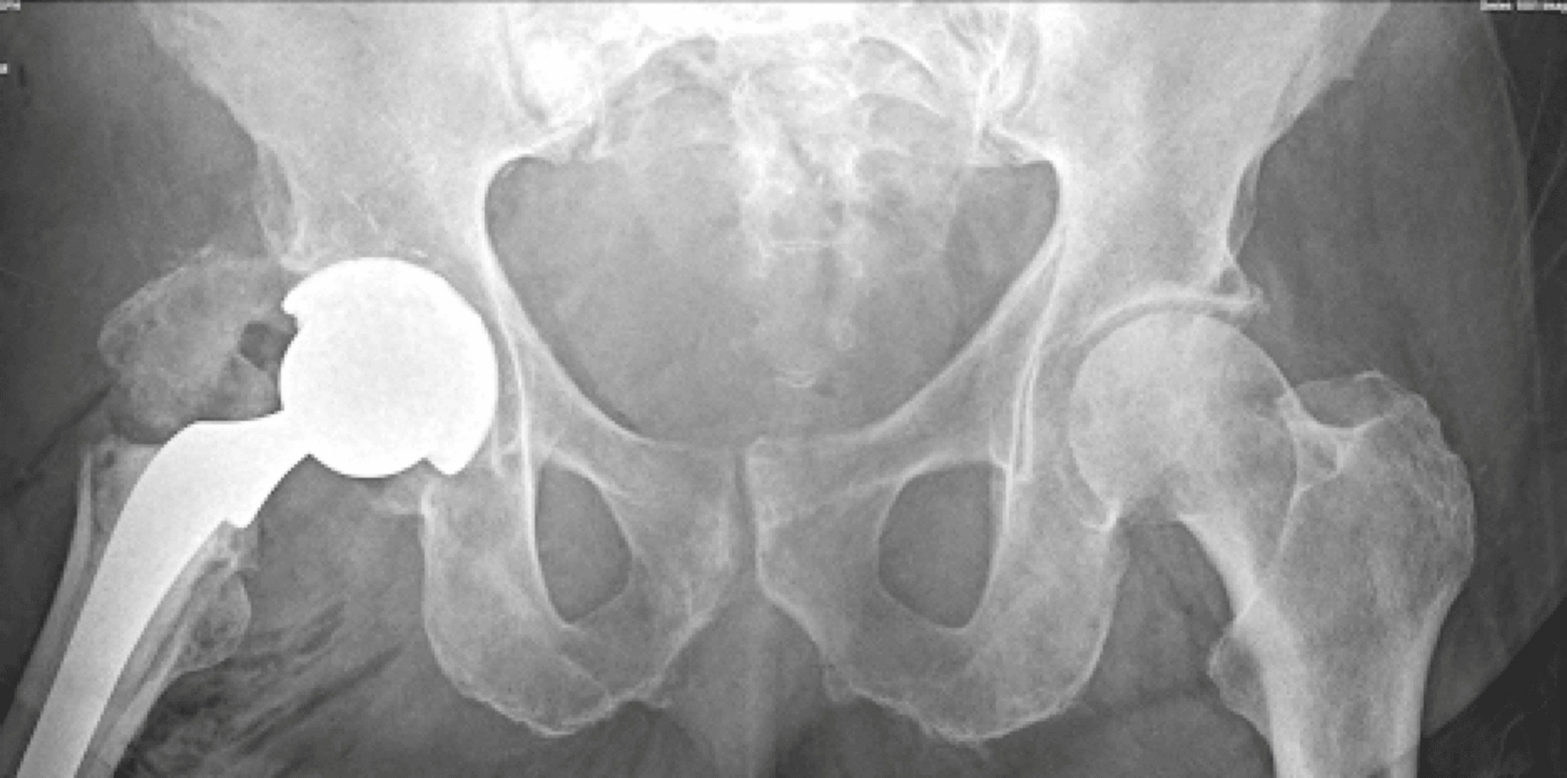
Postoperative anteroposterior radiograph showing intraoperative greater trochanter avulsion. Postoperative AP radiograph showing a displaced greater trochanter fragment resulting from intraoperative avulsion, adjacent to a well-positioned cemented femoral stem.
